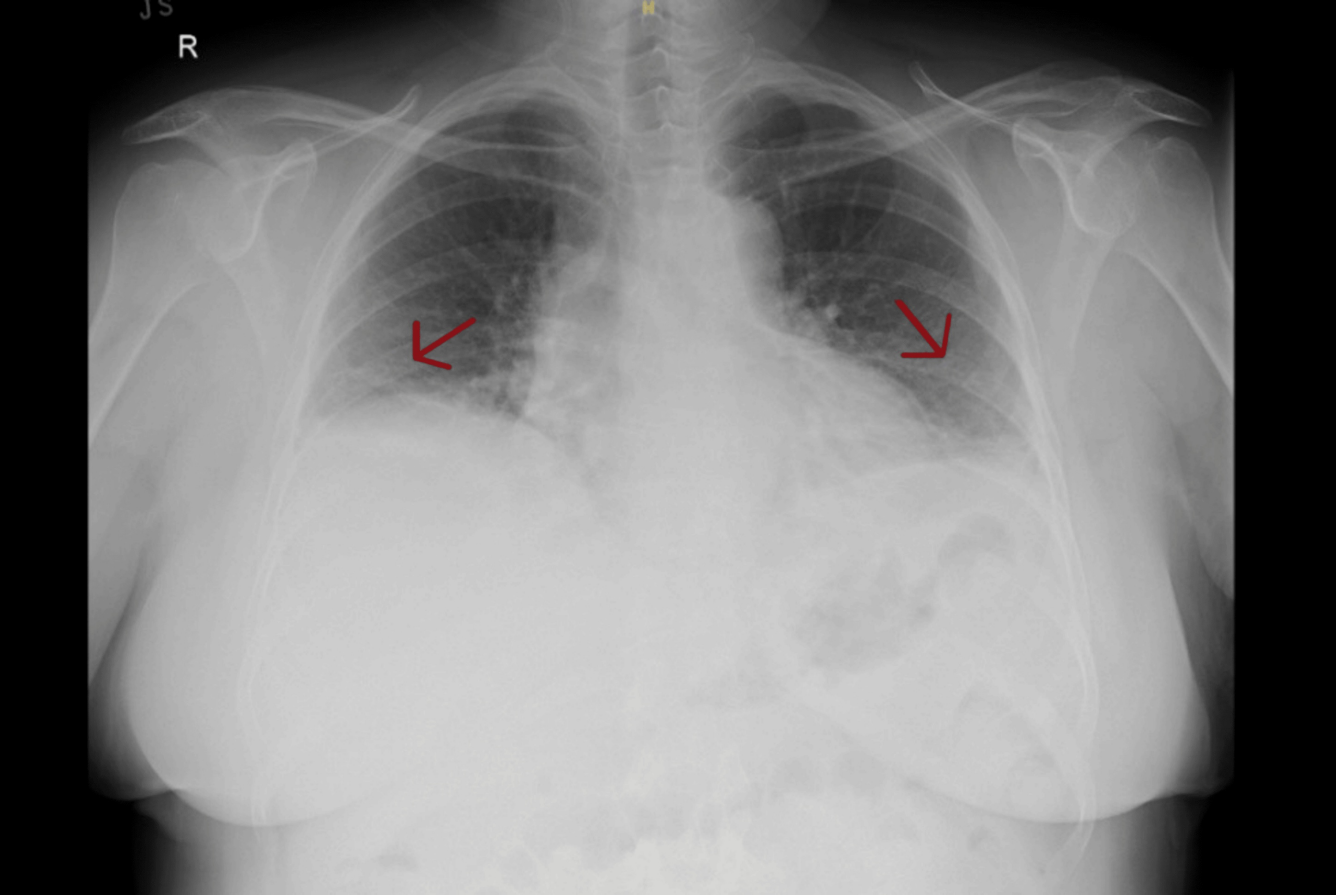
Chest X-ray showing bibasal hazy airspace opacification (red arrows), possibly representing infection An AP rather than a PA view was obtained due to the patient’s severe epigastric pain and shortness of breath, necessitating immediate imaging.
